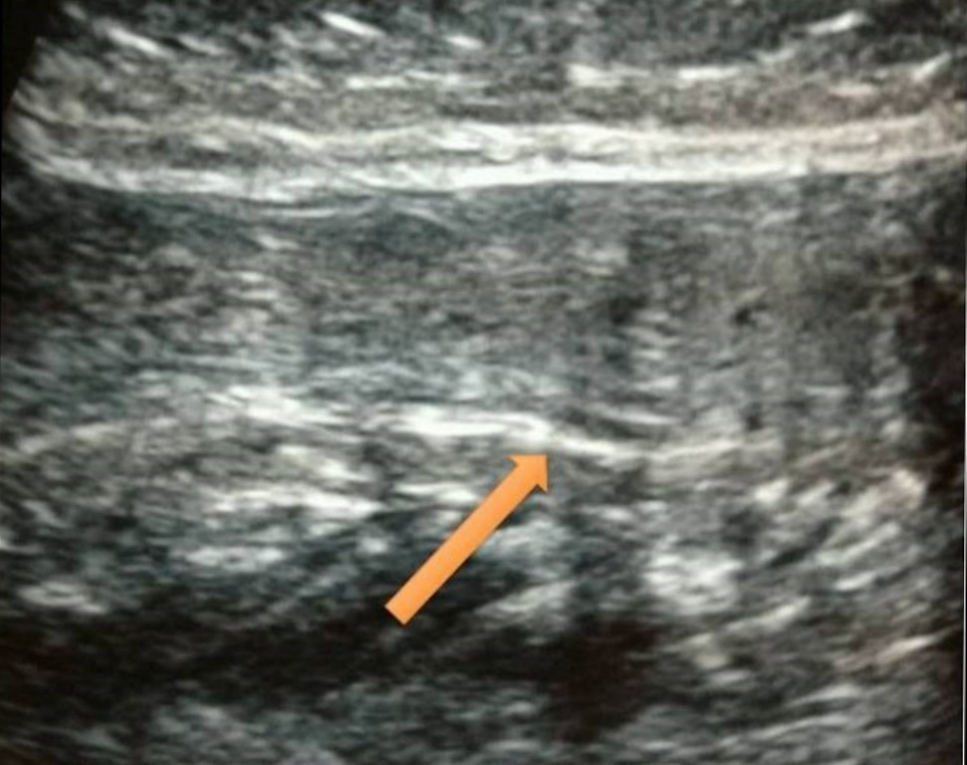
Ultrasonography of left Achilles tendon at nine months follow-up showing complete healing of the tendon.
